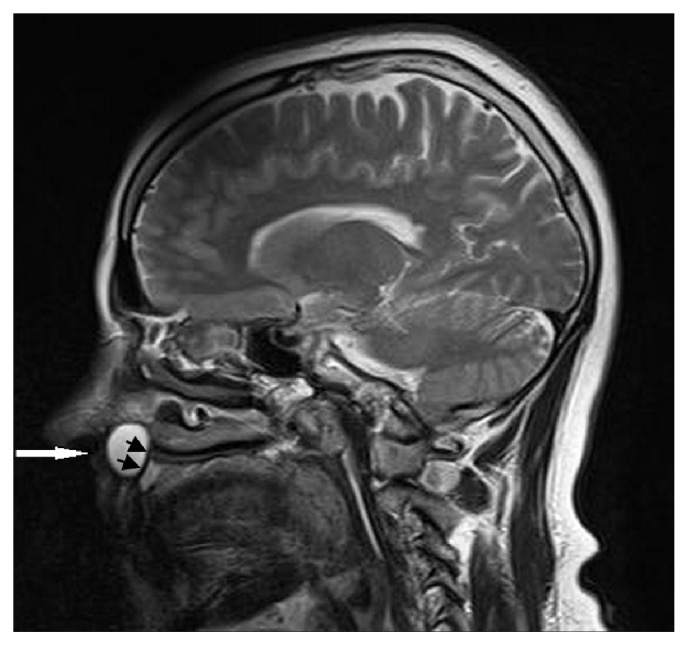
The sagittal T2-weighted MR image shows hyperintense lesion in the lower nasal fossa (white arrow) and bone erosion (black arrows).
